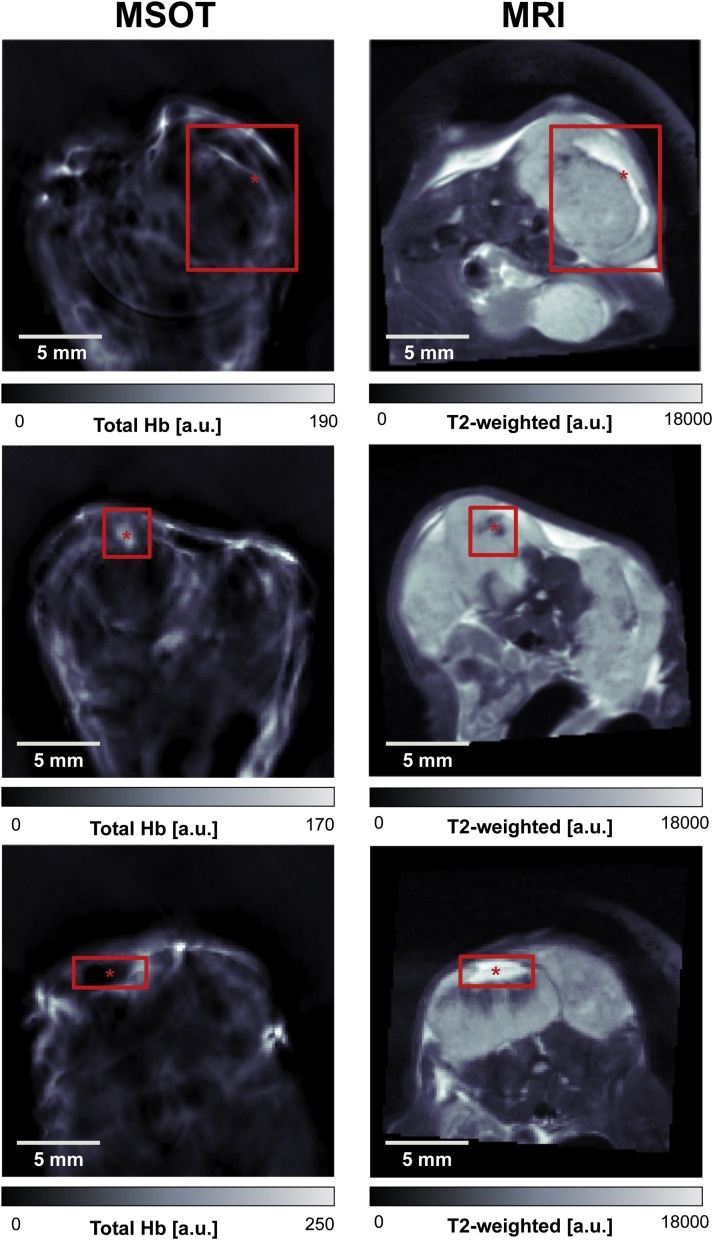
Anatomical features in three K8484 tumours after landmark-based body and tumour contour registration. The red asterisks mark the selected feature centroids by the first operator. Across all observers and tumours, a range of 0.95–6.7 pixels for euclidean distances between MRI and MSOT was calculated. This corresponds to a range from 71 μm to 503 μm in registration error.
